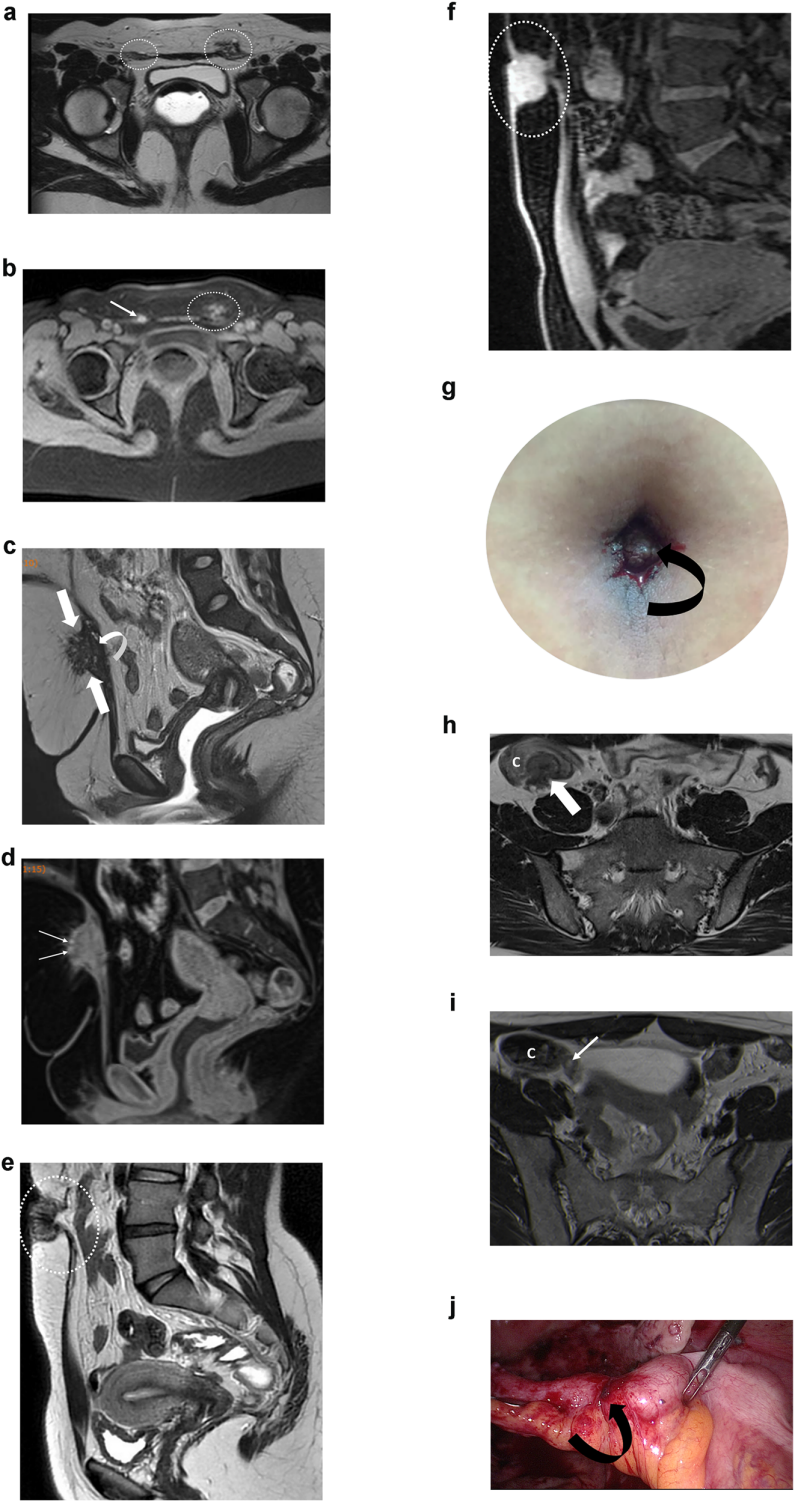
Different examples of extra-pelvic locations of endometriosis. a, b Bilateral inguinal endometriosis in a 31-year-old woman who presented with bilateral inguinal catamenial pain. a Axial T2W MR image shows nodular lesions (areas inside the circles) infiltrating the inguinal portions of the round ligaments. b Axial T1WFS MR image shows high signal intensity foci, likely due to hemorrhagic content (arrow and area inside the circle). c, d Cesarean scar endometriosis in a 40-year-old woman with pelvic pain. c Sagittal T2W MR image shows a nodule (arrows) with heterogeneous signal intensity, irregular margins, and internal areas of high signal intensity infiltrating the subcutaneous tissue and the rectus abdominis muscle (curved arrow). d Sagittal T1WFS MR image shows high signal intensity foci within the nodule, likely due to hemorrhagic content. e–g Umbilical endometriosis in a 31-year-old woman with cyclical bleeding and swelling in the umbilicus. e Sagittal T2W MR image shows a low signal intensity nodule infiltrating the umbilical scar (area inside the circle). f Sagittal T1WFS MR image shows a high signal-intensity umbilical nodule (area inside the oval) due to hemorrhagic content. Physical exam (g) shows an enlarged umbilical scar displaying a wine-colored appearance (curved arrow) associated with active bleeding. h Axial T2W MR image shows a low signal intensity nodule infiltrating the cecum (arrow) in a 32-year-old woman with multifocal pelvic endometriosis. i Axial T2W MR image shows a small nodule attached to the appendiceal base (arrow). Laparoscopic (j) resection confirmed an endometriotic implant (curved arrow). C, cecum
